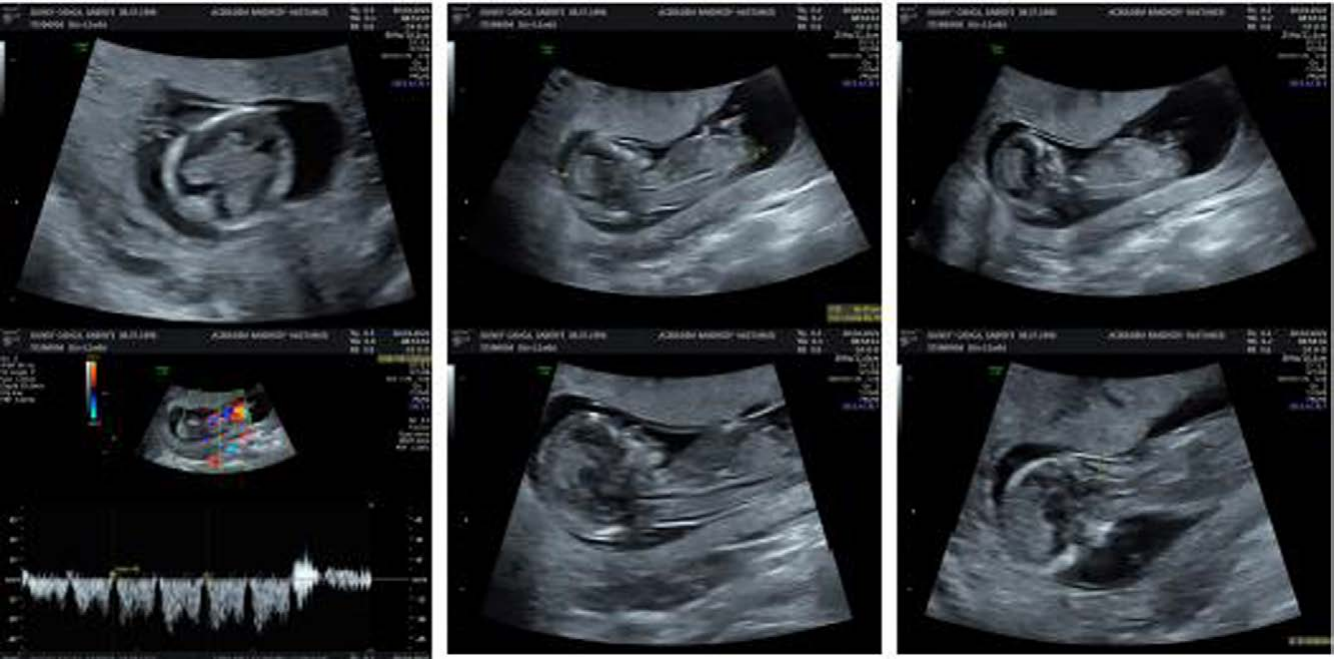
First-trimester ultrasonographic images.
